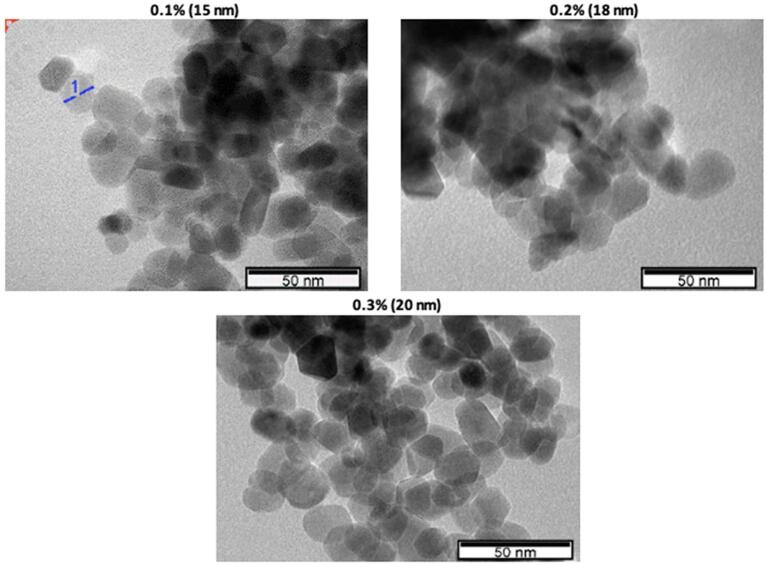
TEM images of ultrasonically untreated ZnO-DW nanofluids.
